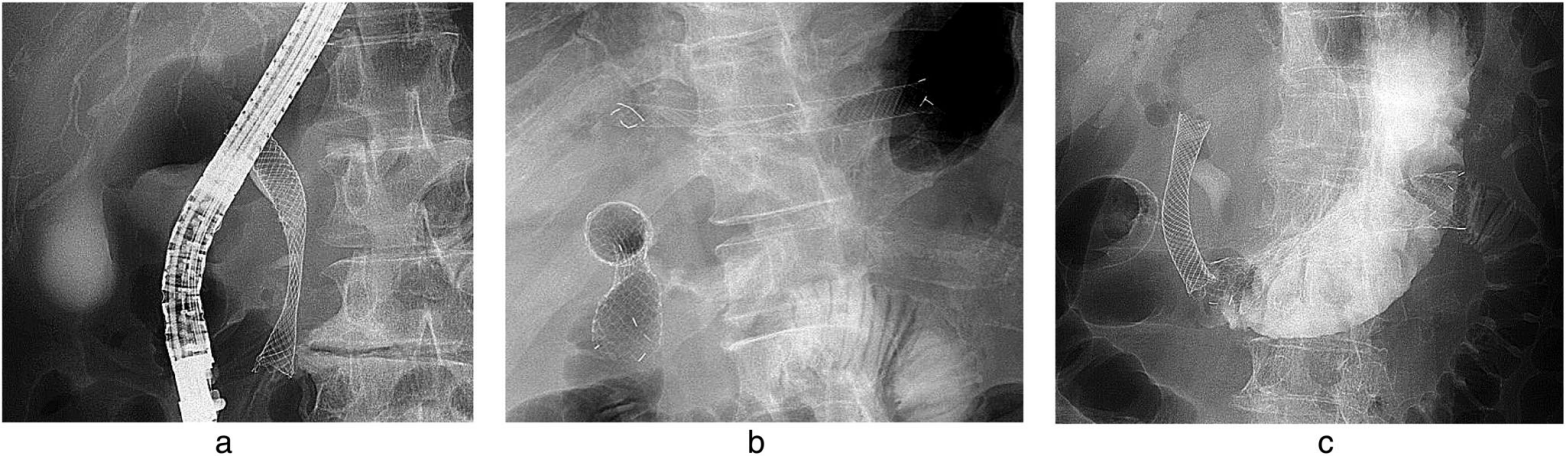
X‐ray images showing different approaches for endoscopic biliary drainage with a metal stent: (a) Endoscopic retrograde cholangiopancreatography‐guided biliary drainage; (b) endoscopic ultrasound‐guided hepaticogastrostomy and a duodenal stent; (c) endoscopic ultrasound‐guided choledocoduodenostomy and a duodenal stent.
